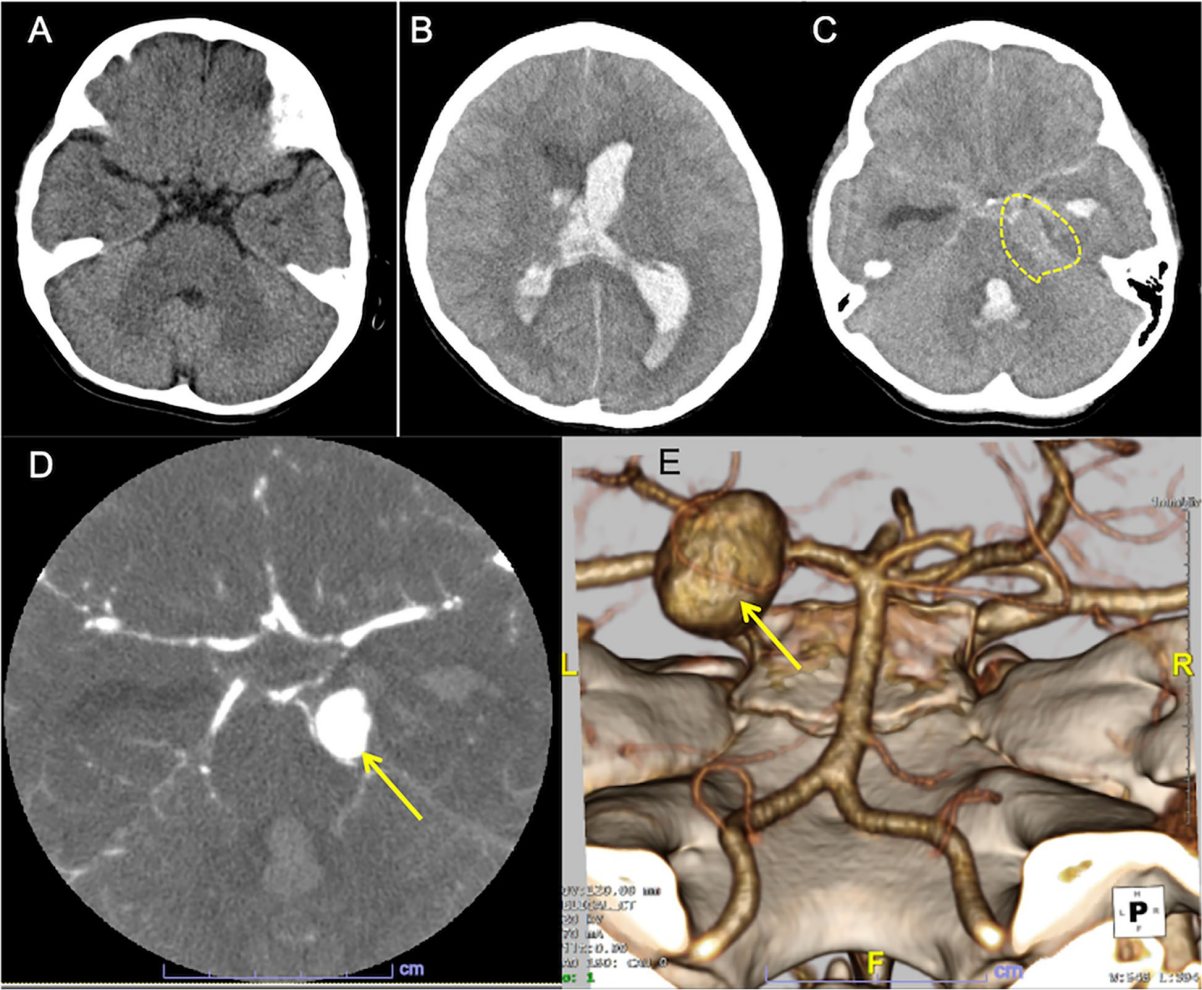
Computed tomography (CT) images. A A CT image taken at age one. No identifiable lesion suggestive of aneurysm. B Axial CT image on admission. The plain CT shows brain swelling, hydrocephalus with intraventricular hemorrhage. C Hyperdense material in the basal cistern, intraventricular hemorrhage in the left lateral and fourth ventricles, as well as a round lesion (encircled) at the left pontocerebellar cistern, indicating intracerebral aneurysm. D CT angiography reveals a large dome-shaped aneurysm (arrow) measuring 15 mm of the left posterior cerebral artery. E 3D-reconstruction image shows a saccular aneurysm in the left P2 segment (arrow)
